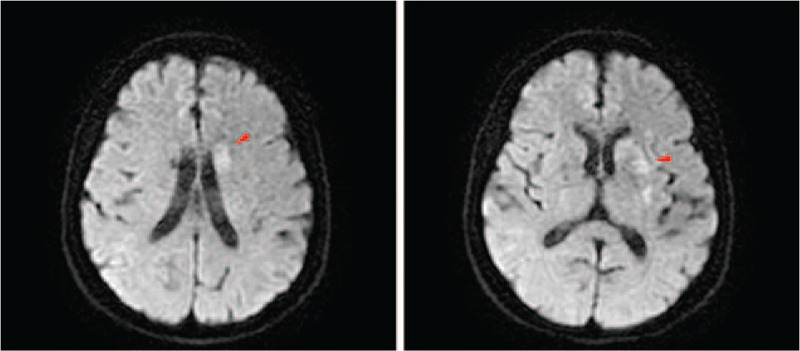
After 24 hours, DWI stimulated acute infarction in the left basal ganglia.
